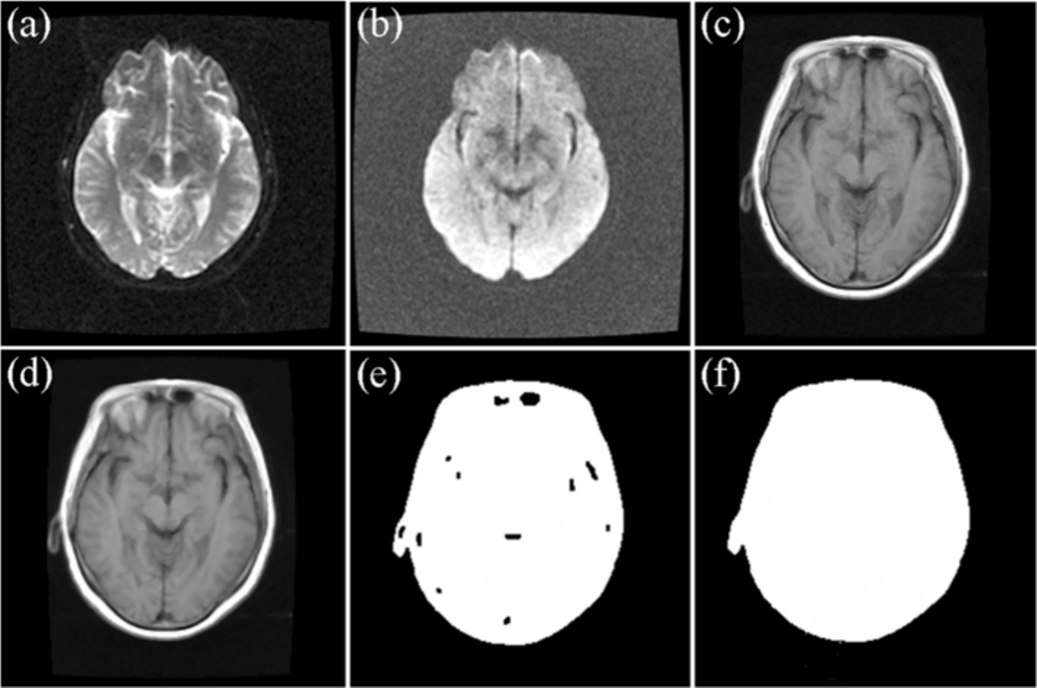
Images of a patient with meningiomais, T2- and PD- weighted MRI are registered to T1 weighted MRI, which is clear and easy to extract background. The background region of T1 weighted MRI is also the background region of registered T2- and PD- weighted MRI. (a) T2 weight MRI, (b) PD weight MRI, and (c) T1 weight MRI, (d) the result of the median filtering for T1 weight MRI, (e) the result of the corresponding morphological close operation, and (f) the final result of the background (dark region).
